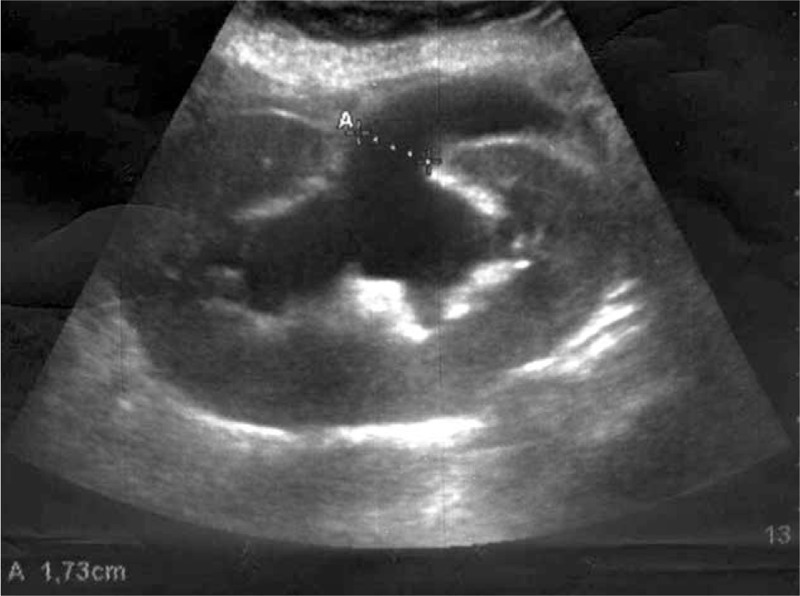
Graft sonography performed 6 h after hernia repair demonstrates severe hydronephrosis.
